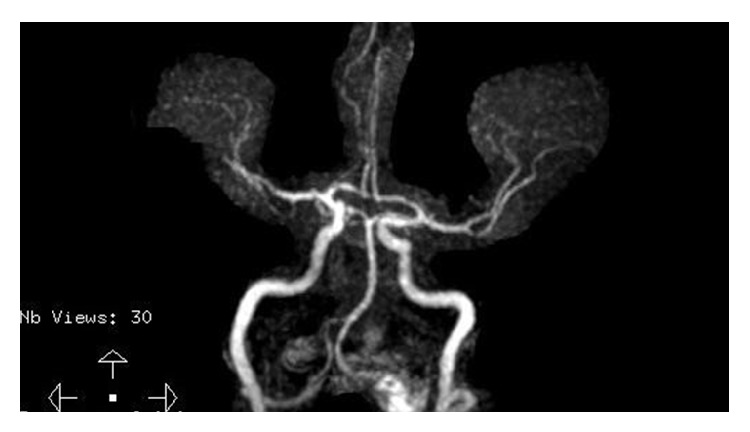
The middle cerebral artery was noted to have recanalized when repeat MRI studies were performed at just beyond 6 weeks postpartum.
